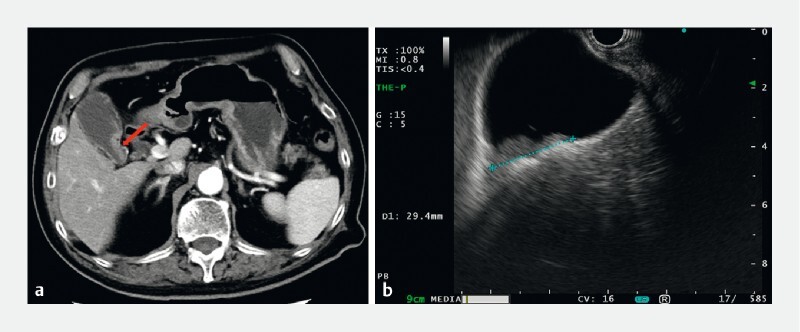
Contrast-enhanced computed tomography showing an enhancing gallbladder mass (arrow).
